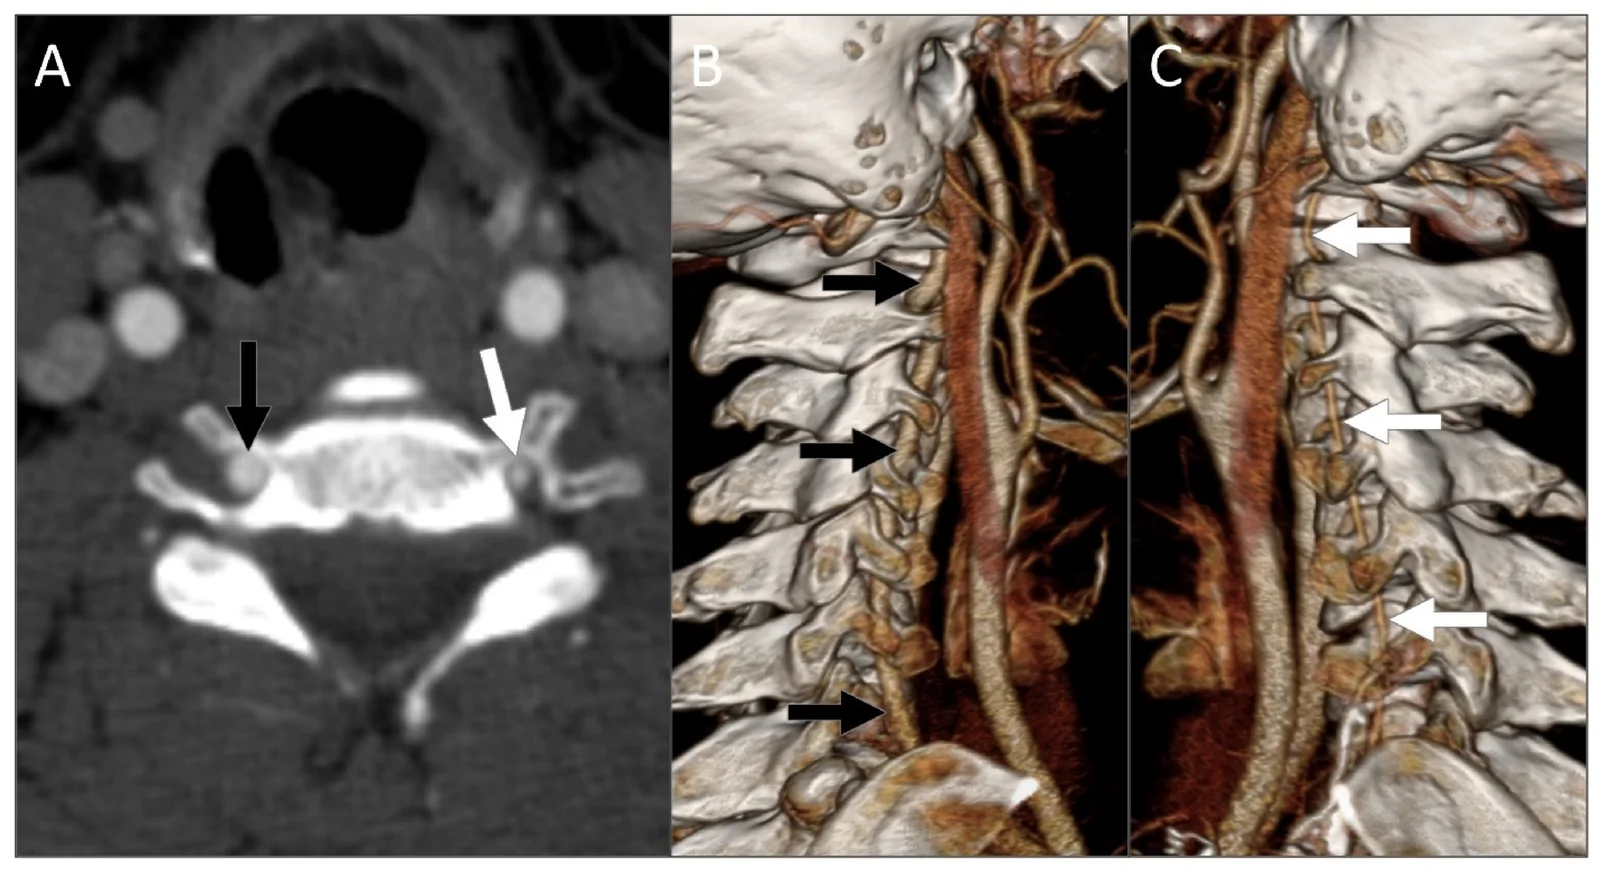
Hypoplastic left VA. Axial CTA (A) and volume-rendered (B,C) images demonstrate an asymmetric, hypoplastic left VA (white arrows) with a dominant right VA (black arrows). The left-sided transverse foramina are also congenitally hypoplastic.
